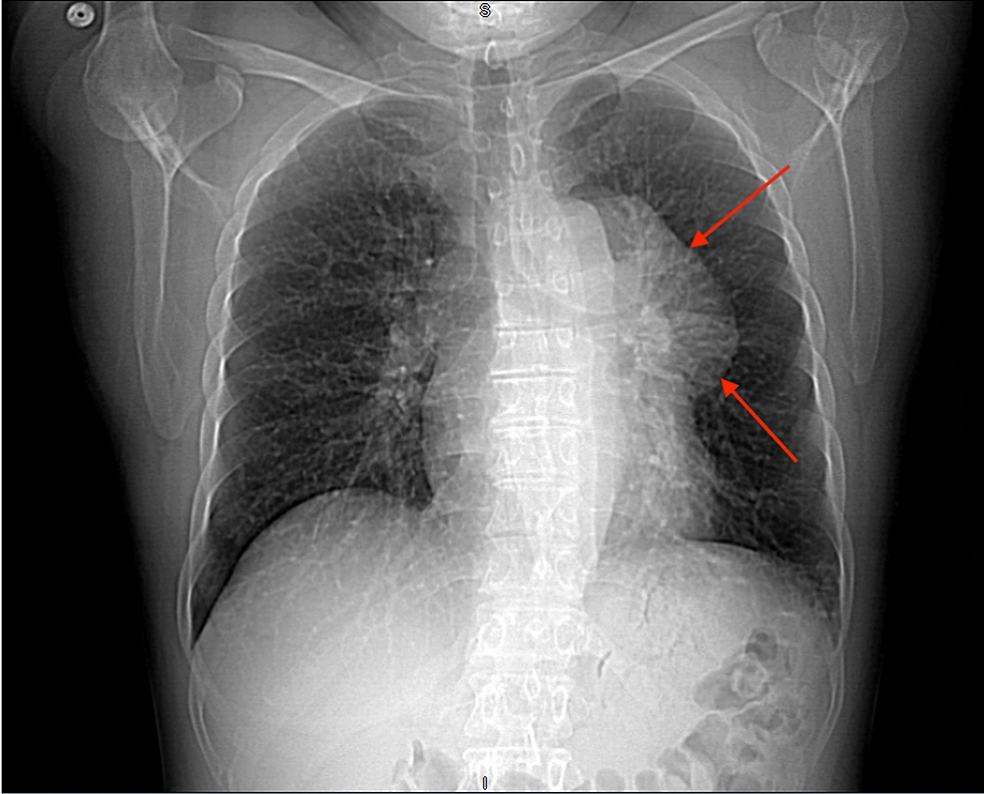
Chest X-ray, anterior view, initial presentation showing anterior mediastinal mass which extends into the left hilum
